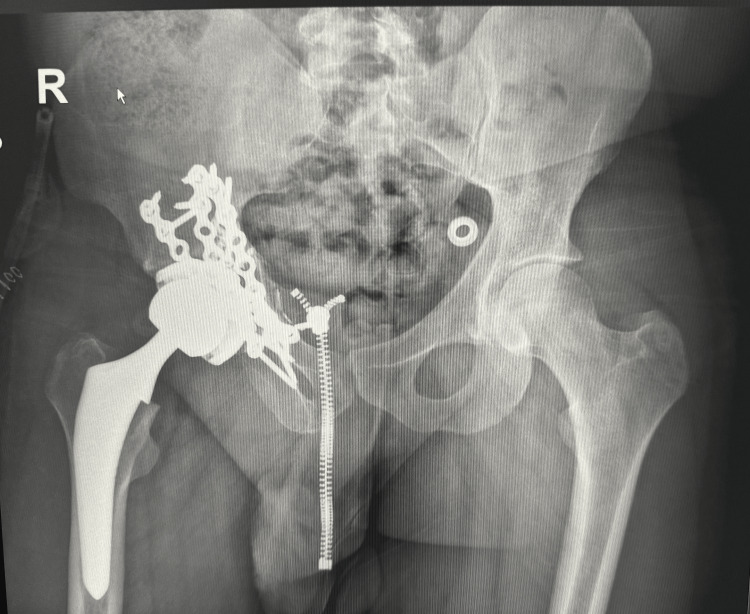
Postoperative AP pelvic X-ray of primary total hip arthroplasty AP, anteroposterior
